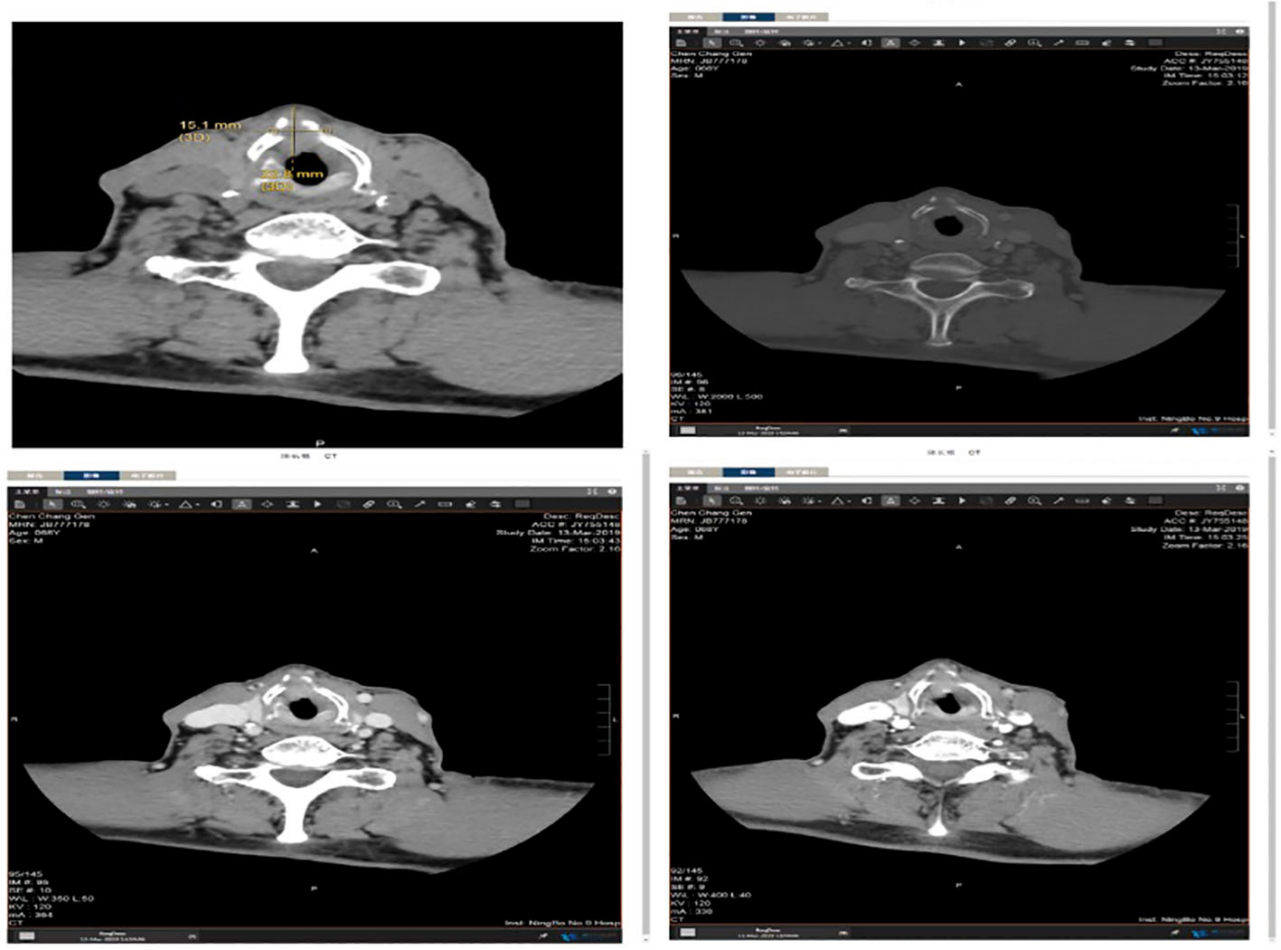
Larynx tomography (CT) scan showing that the morphology of the right vocal cord is fixed with thickening, about 6mm, extending forward to the anterior commissure of the vocal cord and the anterior edge of the left vocal cord.
